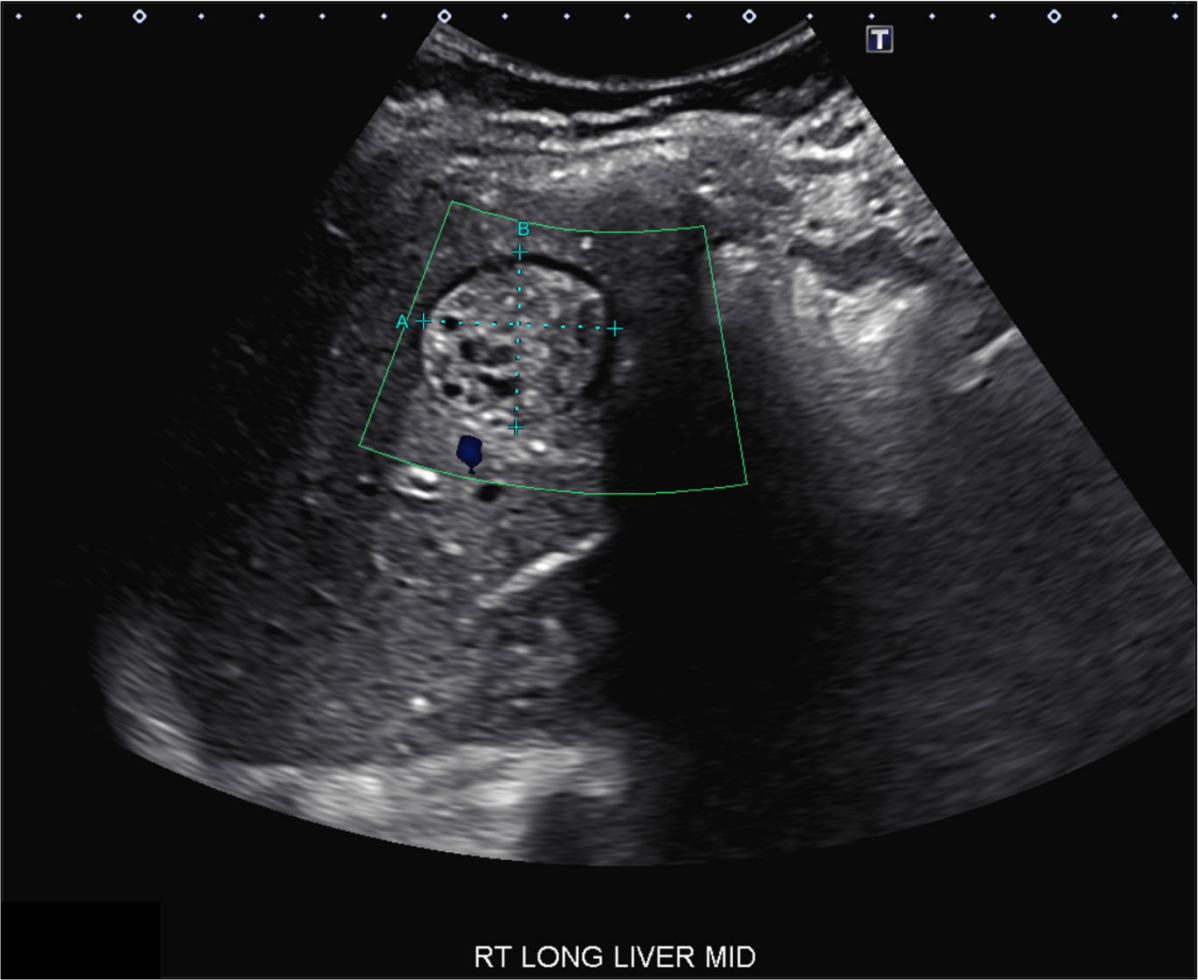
Sagittal color Doppler ultrasound image of the right hepatic lobe demonstrates a 3.1 (A) x 2.9 (B) cm avascular mixed hyper- and hypoechoic lesion with intracystic components.
